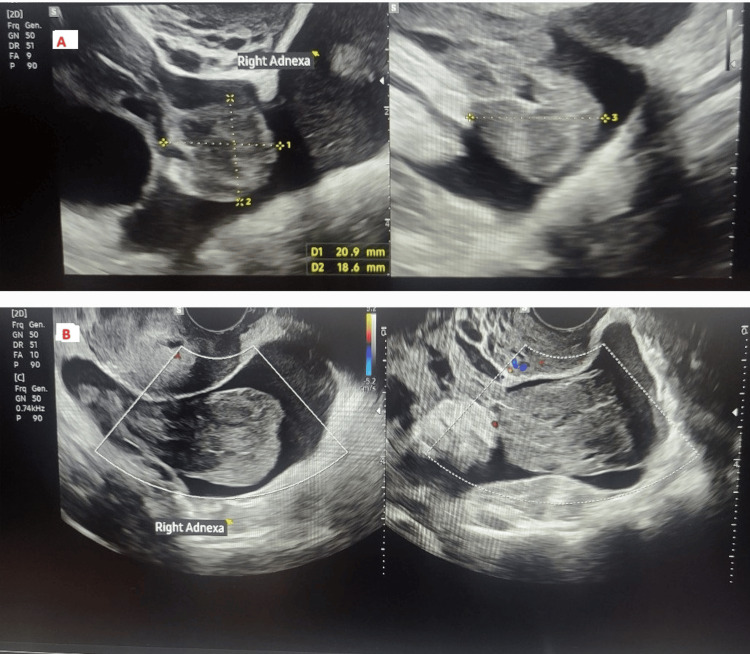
Transvaginal ultrasound demonstrated an inhomogeneous ectopic mass (A) in the sagittal and (B) coronal plane. Inhomogeneous, hyperechoic mass measuring 20.9 x 18.6 x 19.1 mm adjacent to the right ovary, lacking internal vascularity on colour Doppler.
